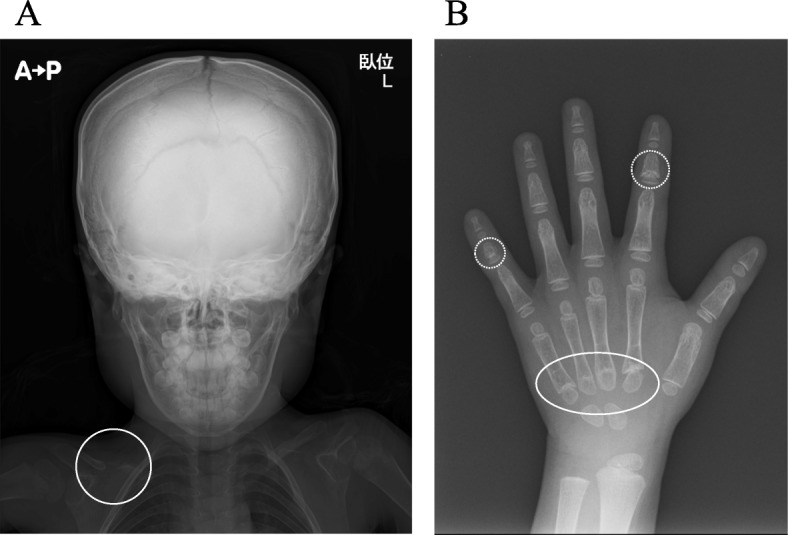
Characteristic findings in the CCD patient. a Skull X-ray shows an open anterior fontanelle and hypoplasia of the right clavicle (white circle). b Wrist X-ray image shows hypoplasia of the middle phalanges (white ellipse) and pseudoepiphyses of the second and fifth metacarpals (dotted circles)
